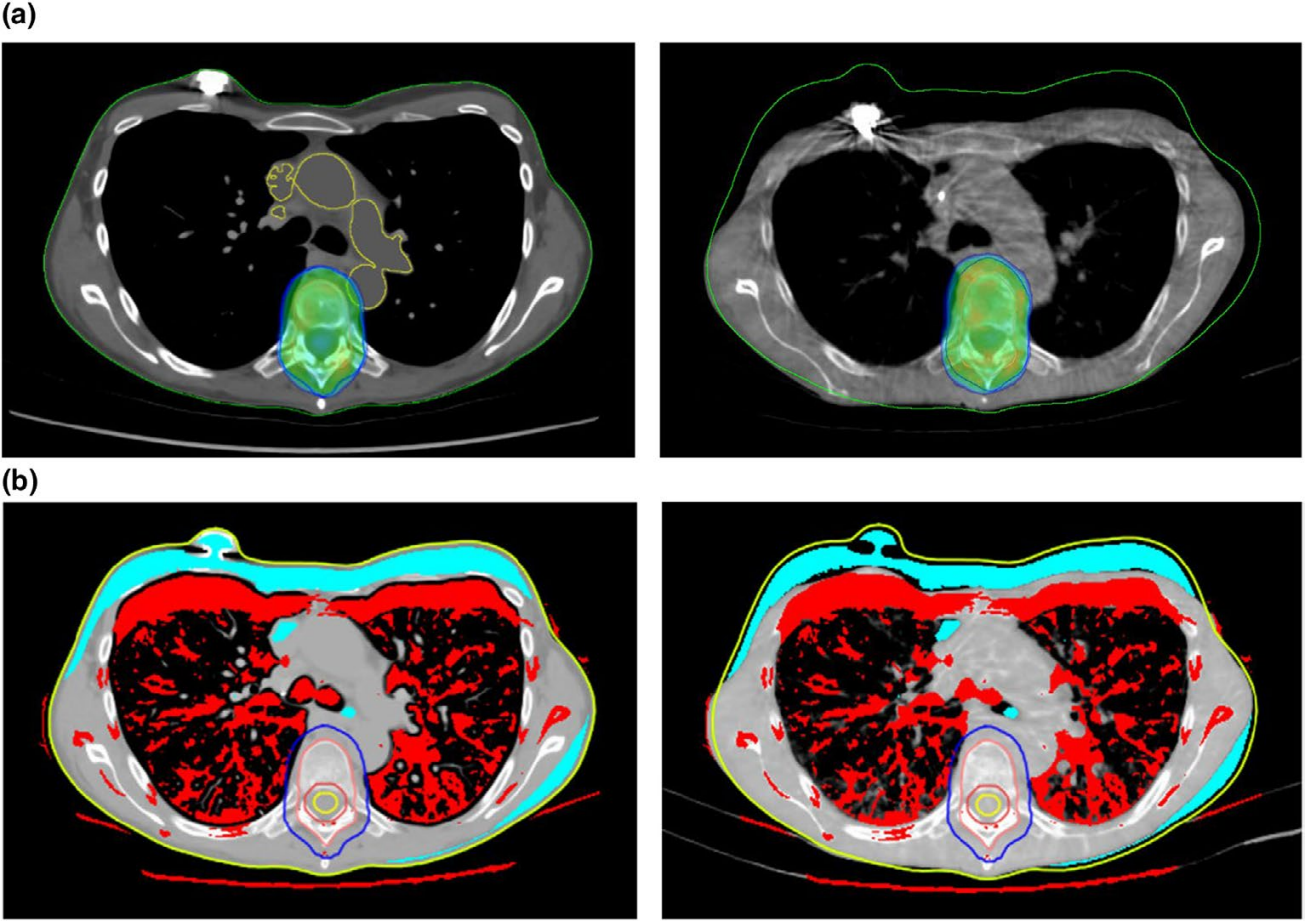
(a) An example of colour wash dose distributions on dCT (left) and dose dump on CBCT (right). The dose range displayed is 90%–110% of the target dose, in this case 8Gy. Contrast has been contoured and forced to a soft tissue density in the dCT plan. Note the differences in external contour and other anatomy on the CBCT plan, but the relatively similar dose distributions. (b) An example of Mobius CT data matches with the dCT (left) and CBCT (right). The external and target contours are both from the original dCT plan. Note the representation of differences in CT values.
